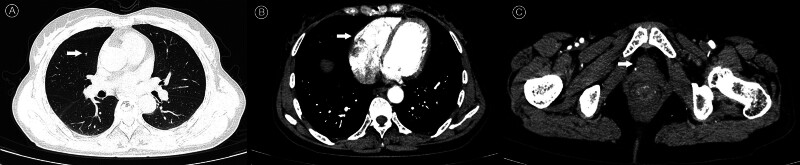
Posttreatment imaging evaluation of pulmonary, cardiac, and urethral involvement following 6 cycles of “paclitaxel + carboplatin + tislelizumab” therapy (November 12, 2024). (A) Chest CT demonstrates marked regression of the pulmonary metastasis (arrow). (B) Cardiac CT shows near-total resolution of the myocardial metastasis (arrow). (C) Pelvic CT shows resolution of the urethral metastatic focus (arrow) with residual periturethral stranding. CT = computed tomography.
